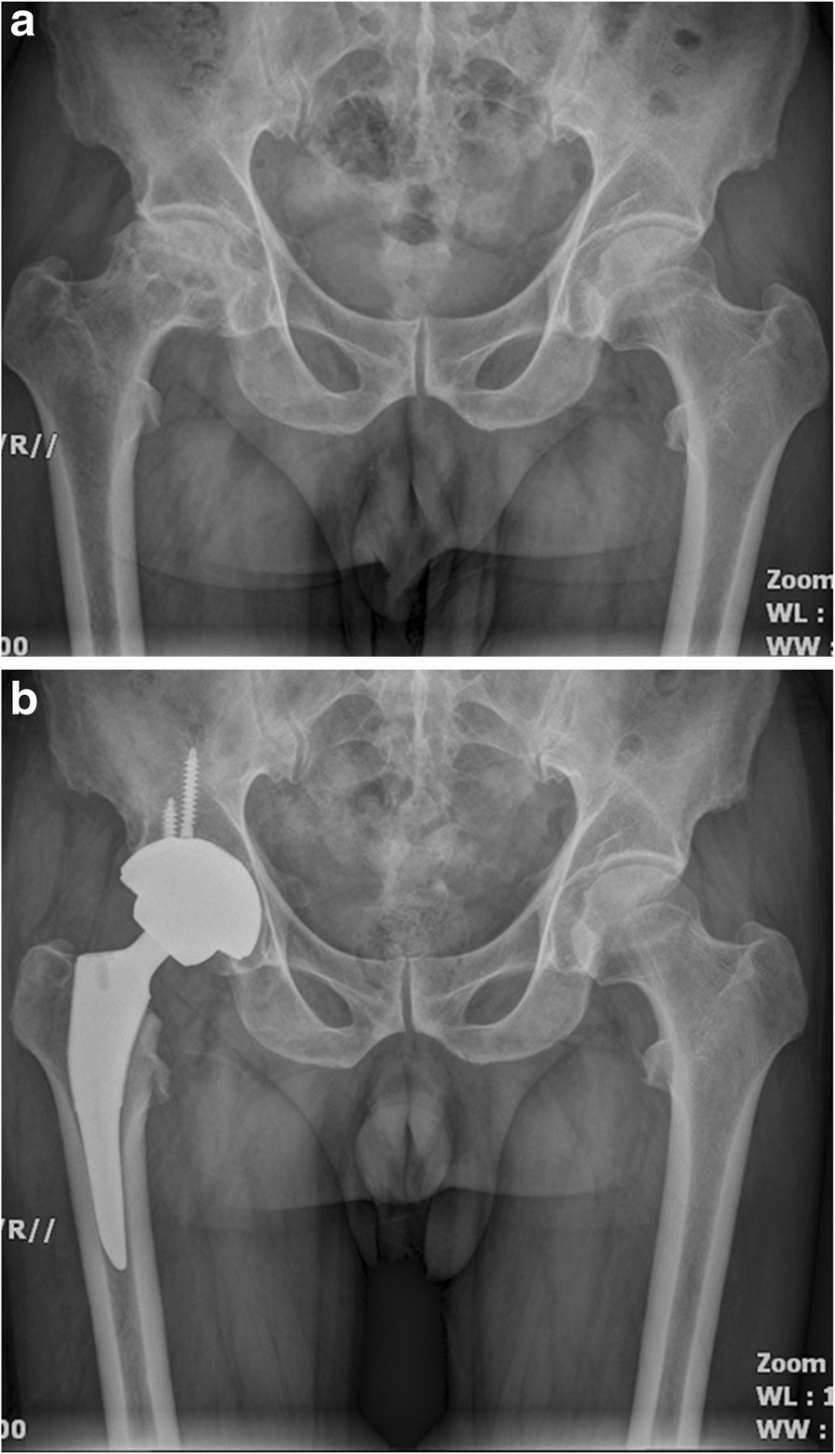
a A preoperative radiograph of the hip of a 75-year-old man who had femoral head osteonecrosis. b A radiograph at 28 months after implantation of short cementless femoral stem showed solid fixation in a satisfactory position
